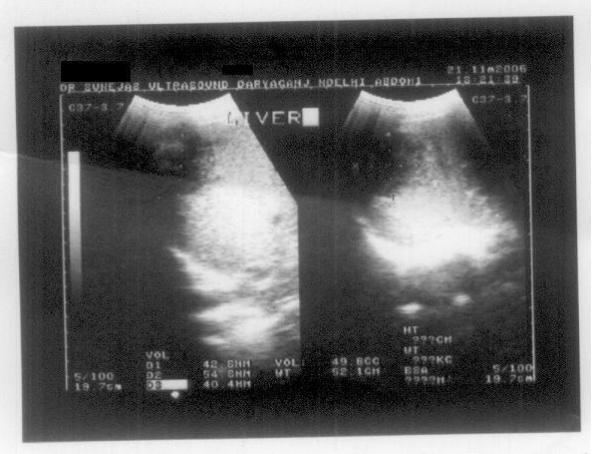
Ultrasonogram of the liver showing a hypo-echoic lesion taken when the patient presented to the outpatient department for the first time.
